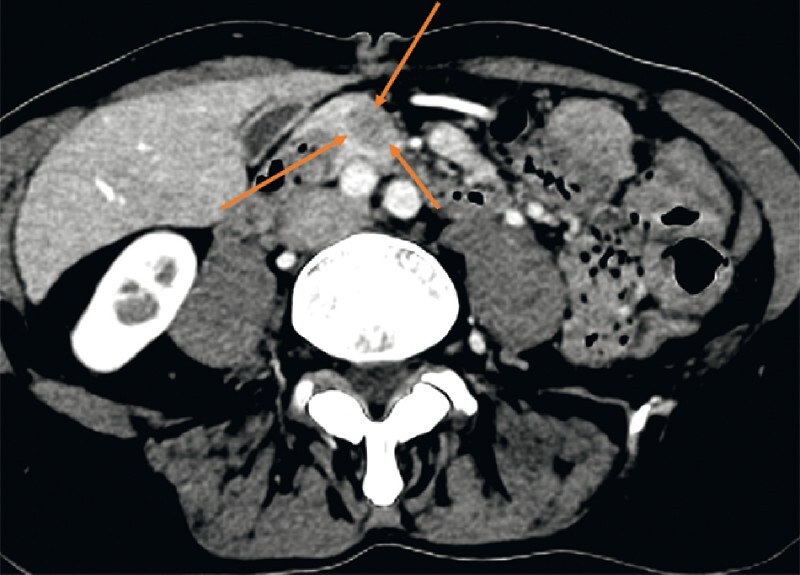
Computed tomography scan showing hypodense pancreatic head lesion (red arrow).
